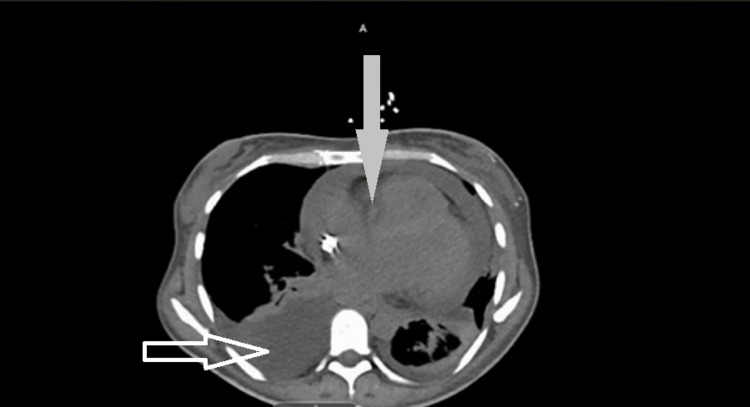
CT of the chest without contrast (axial view) demonstrating a moderate pericardial effusion (solid white arrow) and a left-sided pleural effusion (transparent white arrow)
